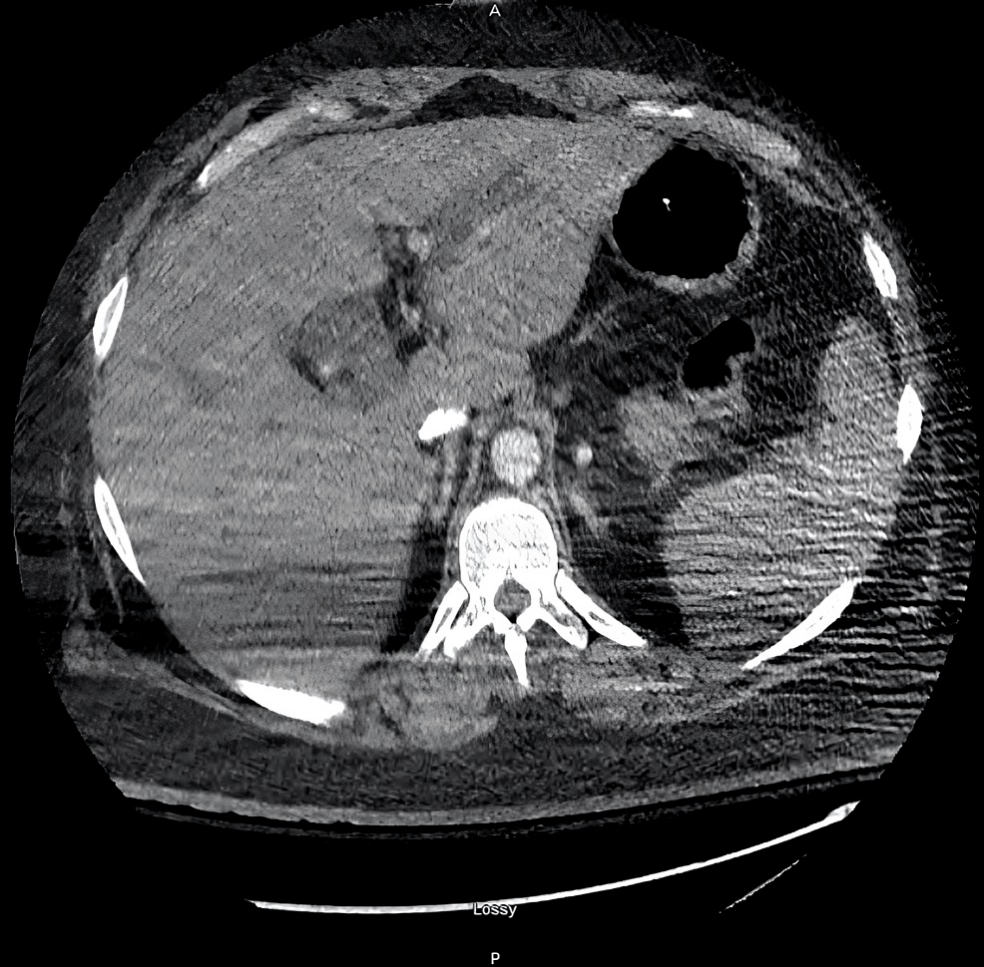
CTA pulmonary showing hepatosplenomegaly CTA: computed tomography angiography
